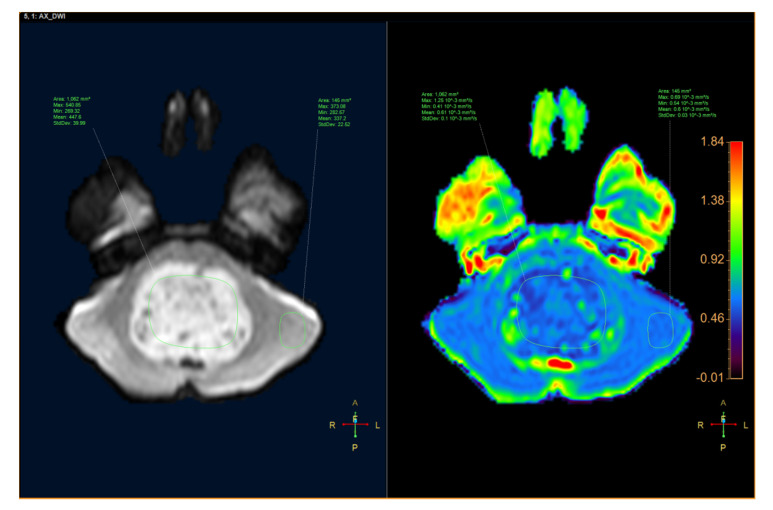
An 8-year-old male patient had a tumor inside the fourth ventricle, which was confirmed as medulloblastoma after surgery. (Left) Axial diffusion-weighted image (DWI). (Right) apparent diffusion coefficient (ADC) map.
